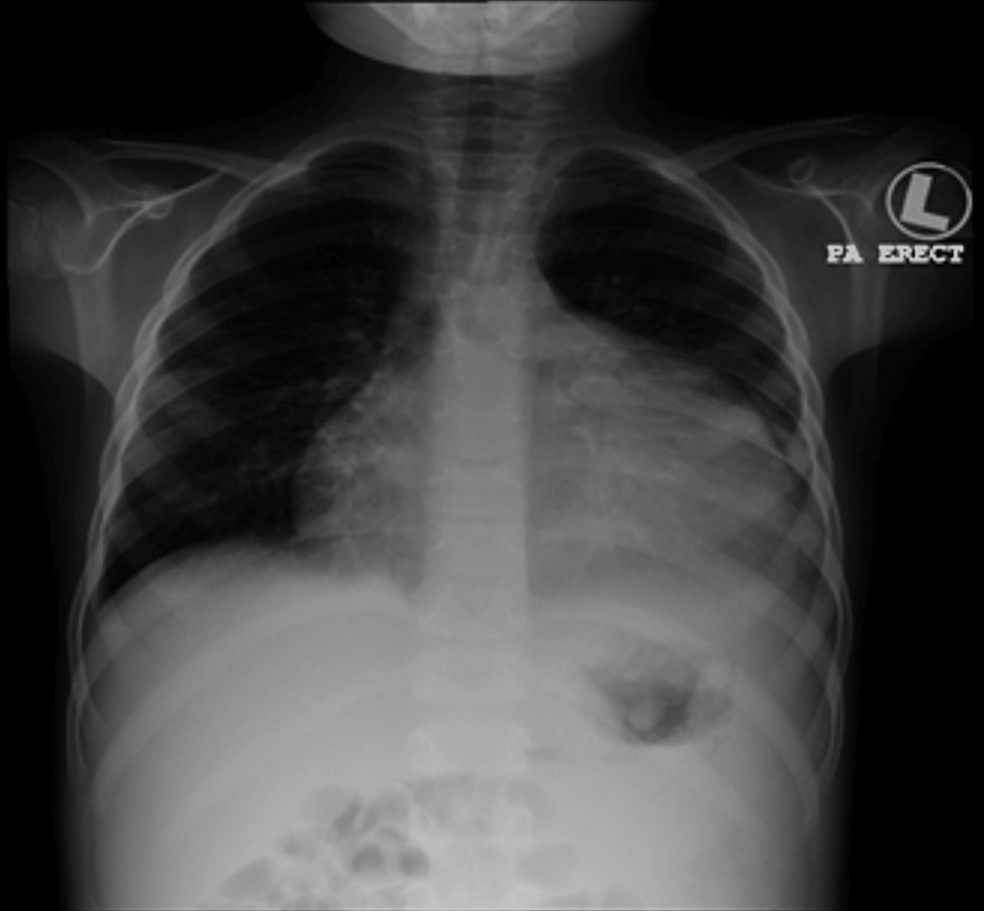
Chest X-ray showing cardiomegaly
